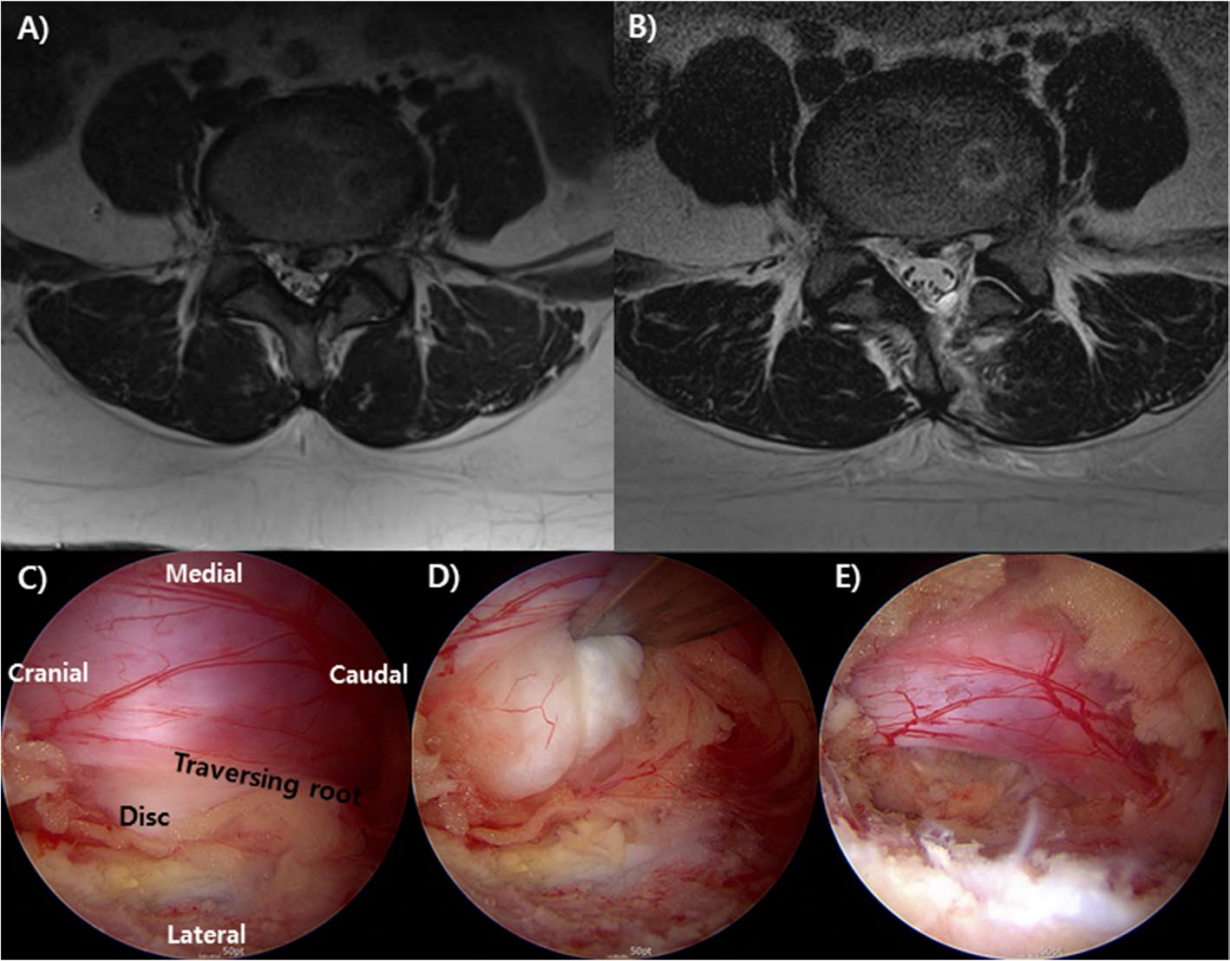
A 32-year-old woman with severe lower back and left lower extremity radiating pain. The patient’s body mass index was 37.8 kg/m^2^. A The preoperative T2 axial magnetic resonance image (MRI) shows a huge disc compressing the thecal sac and nerve root. B The postoperative T2 axial MRI shows the well-decompressed thecal sac and nerve root. Intraoperative endoscopic findings (C) before discectomy, (D) during discectomy, and (E) after discectomy. Huge pieces of disc material were removed, and the thecal sac and nerve root were freely movable after discectomy
